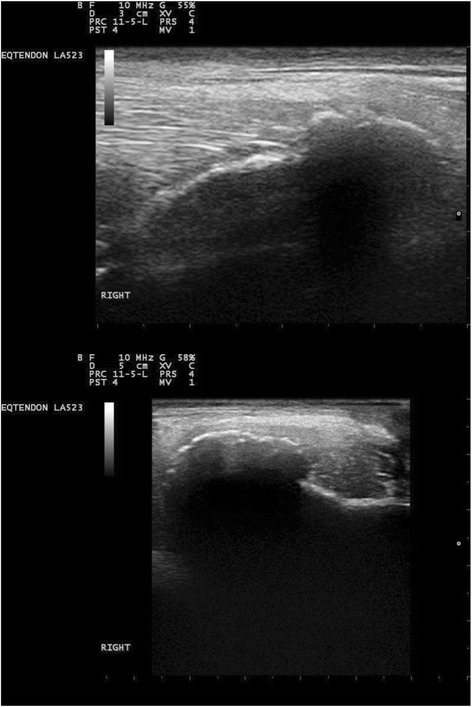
Ultrasonographic image of the lateral aspect of the elbow of a 3 year old thoroughbred gelding. Longitudinal and transverse images of the lateral epicondyle of the right elbow. There is an irregular bone surface and remodelling of the lateral epicondyle of the right humerus at the site of the origin of the ulnaris lateralis tendon. The fibre pattern of the ulnaris lateralis tendon was disrupted on the longitudinal view, with a discrete hypo-echoic region at the site of origin onto the lateral epicondyle
